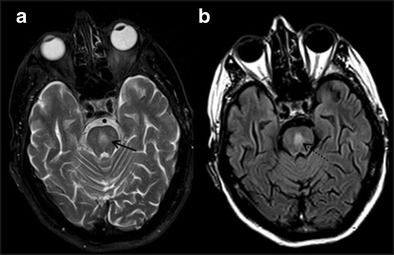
Central Pontine Myelinolysis (CPM). A 61-year-old male with history of ETOH abuse with decrease in function, poor balance and dementia. a & b axial T2W and FLAIR images at the level of pons show central pontine hyperintensity (*black arrow*)
